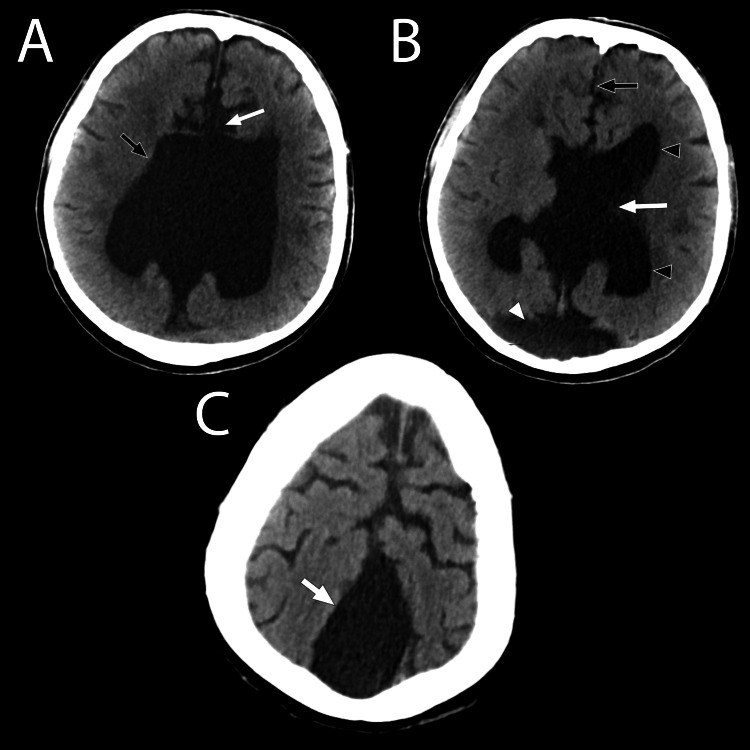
Axial CT series of the brain at various levels A, B, and C. (A) The monoventricle (black arrow) and absence of the corpus callosum (white arrow) are appreciated in this view. (B) The monoventricle (white arrow) and the posterior fossa cyst (white arrowhead) of the Dandy-Walker malformation are demonstrated in this view. The anterior portion of the longitudinal fissure (black arrow) depicts clear non-fusion of the frontal lobes. The frontal horns and trigone of the lateral ventricular components of the monoventricle can be visualized at the level (black arrowheads). (C) White arrow demonstrating the dorsal, midline interhemispheric cyst
